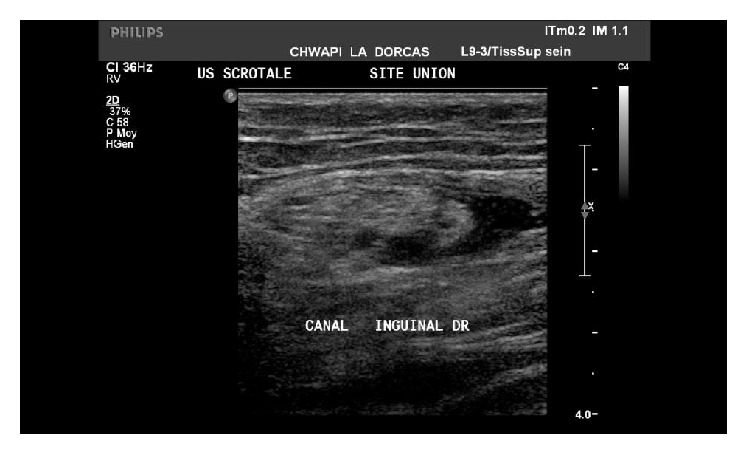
Ultrasound scan demonstrating an infiltration of the inguinal canal fat.
